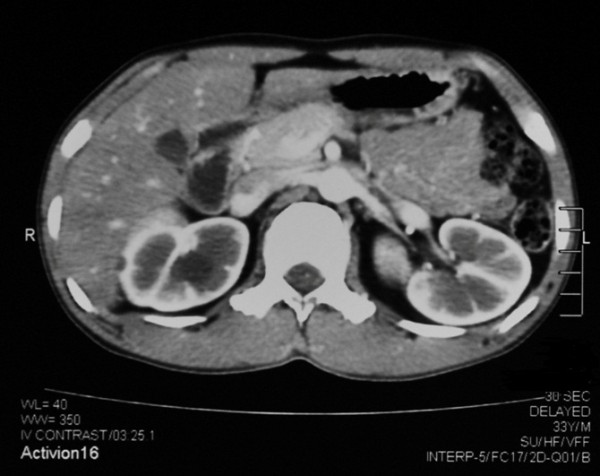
Contrast-enhanced CT scan abdomen showing multiple medullary cysts in both kidneys.
